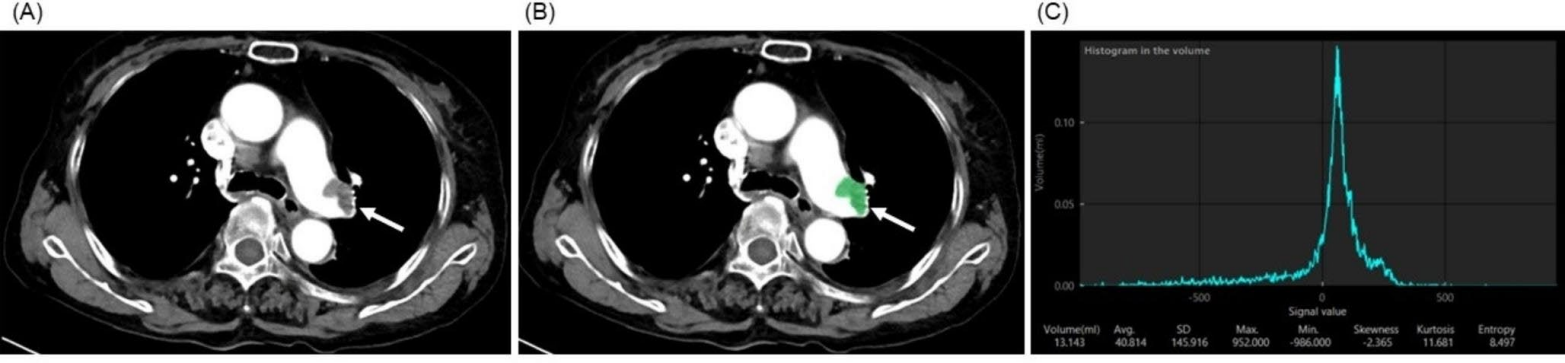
Methods of measuring clot volume using CT. (A) CT shows thrombus in left pulmonary artery (arrow). (B) Manually select the range of thrombus (arrow) in each slice. (C) The workstation automatically calculated the pulmonary embolus volume
